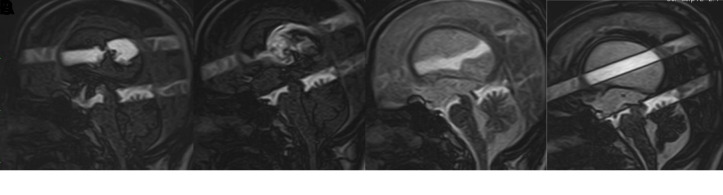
Magnetic resonance imaging (MRI) real-time time spatial inversion pulse (SLIP) image. Cerebrospinal fluid in the body of lateral ventricle was stirred by inertia (head shaken). Cerebrospinal fluid agitation become settled 3 and a half minutes after head is shaken. In the middle of head shaking (A), after 1 minute (B), after 2 minutes (C), and after 3 and a half minutes (D).
